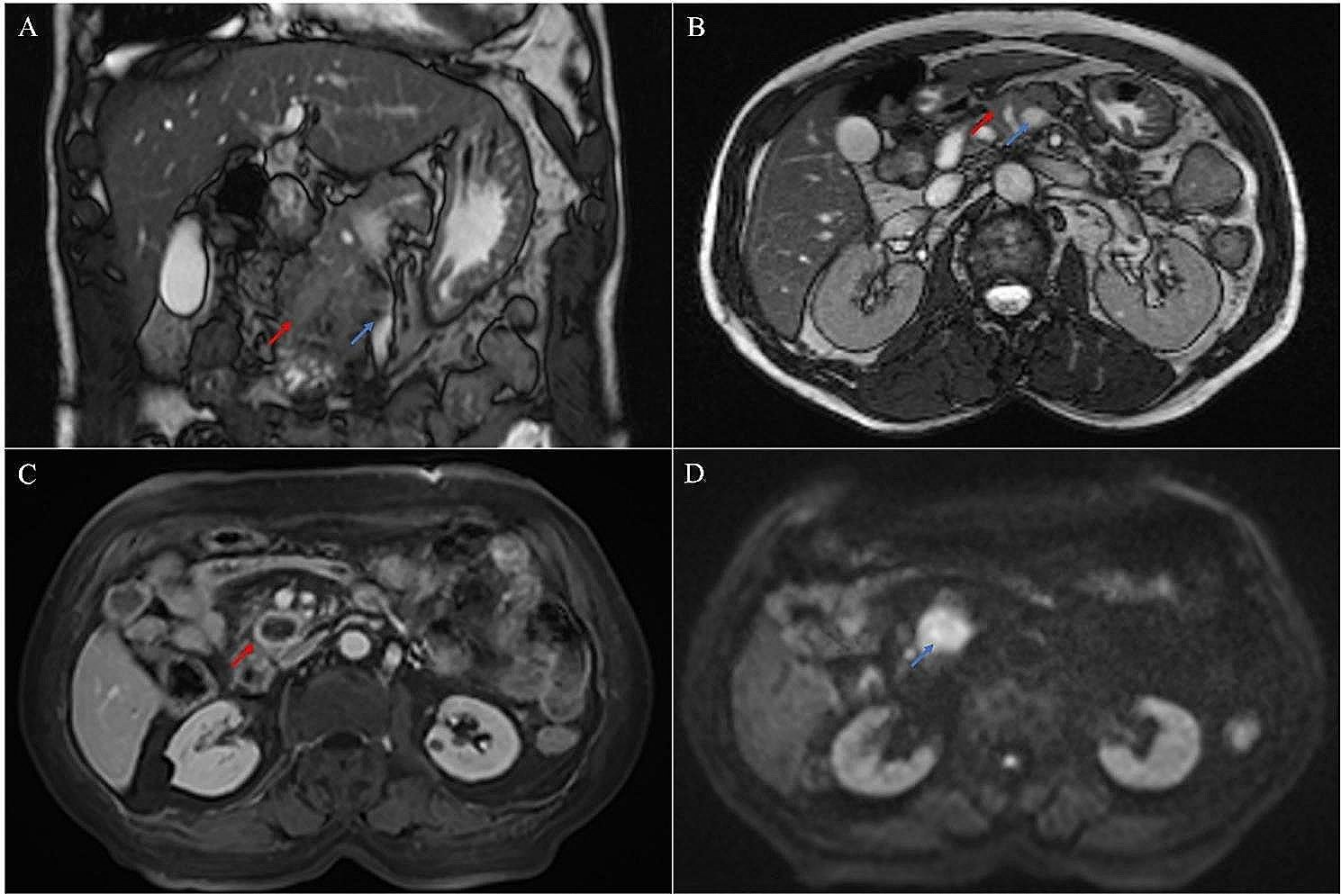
MRI scan of the abdomen. (A, B). A 71-year-old male patient presented with a low-density tumor located in the uncinate process of the pancreas with ill-defined margins, heterogenous enhancement on enhanced scans (red arrow), and the SMV was encased by the tumor (blue arrow). (C, D). An 80-year-old male patient presented with a low-density ill-defined margins tumor located on the head of the pancreas, T1WI showed circular enhancement (red arrow), and DWI showed restricted diffusion (blue arrow)
